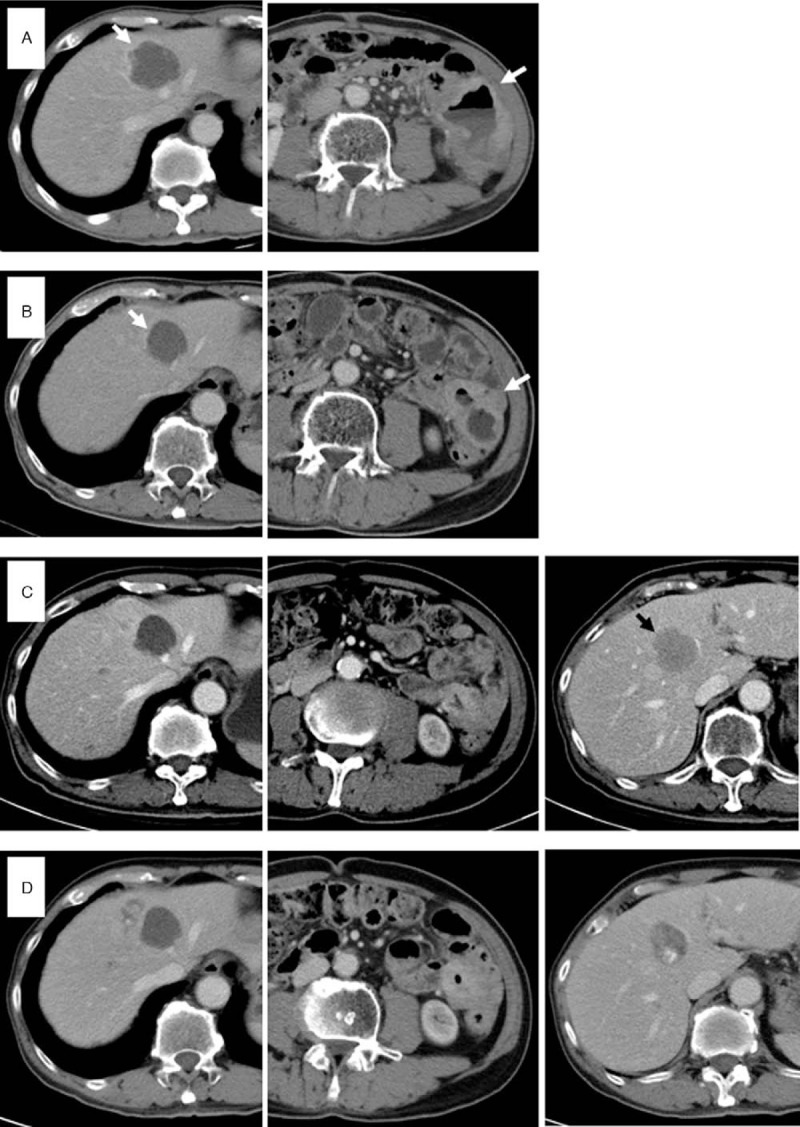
Abdominal computed tomographic images following local treatments for metastatic lesions and systemic trifluridine/tipiracil administration. The images display the tumors following local therapies of radiotherapy and Yttrium-90 radioembolization (A), as well as 4 (B), 7 (C), and 10 (D) months after initiating trifluridine/tipiracil administration. Arrows indicate tumors with a decrease in size and black arrow indicates a new lesion.
